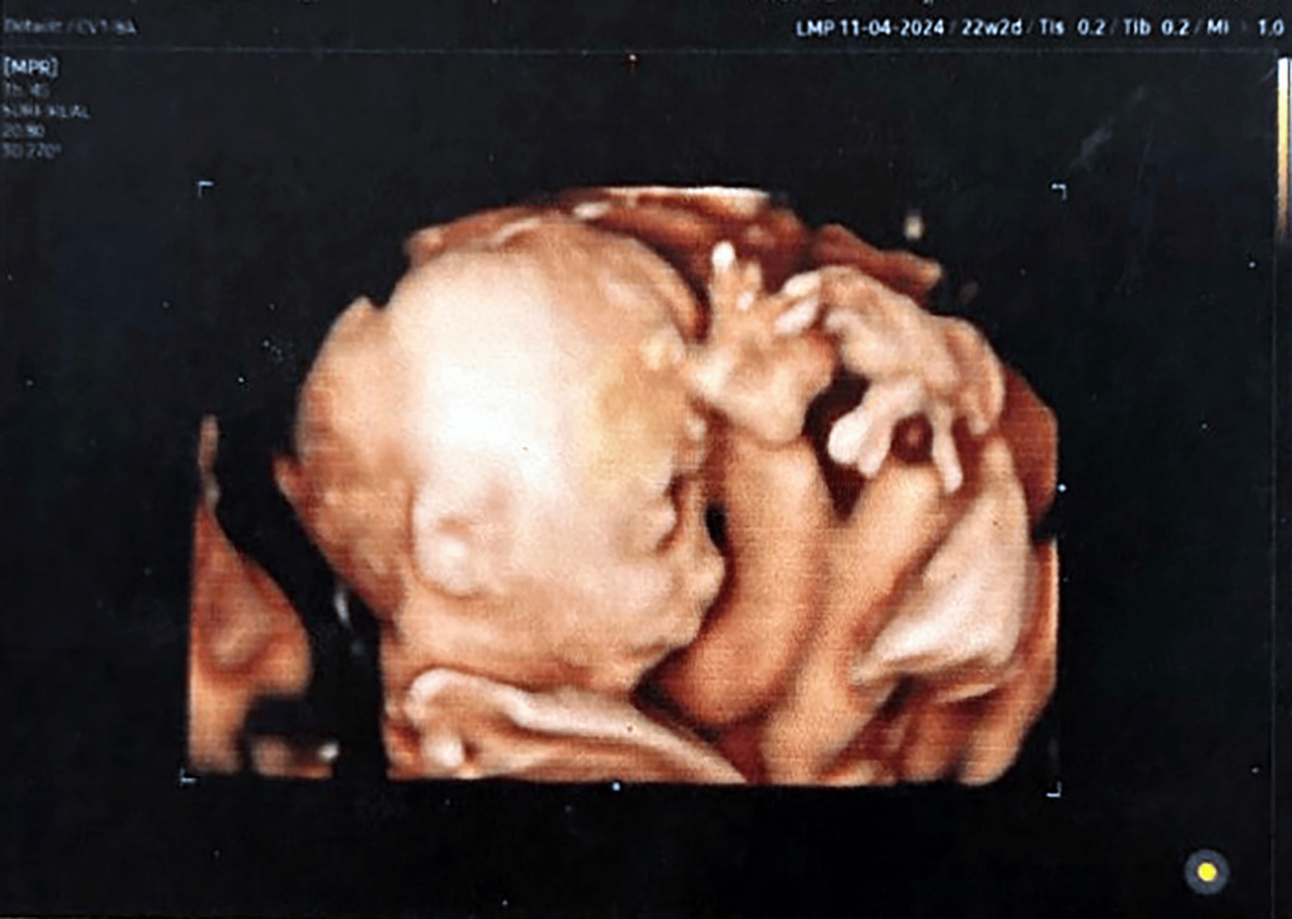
3D/4D Ultrasound scan at 22 weeks of gestation showing no structural anomalies.
